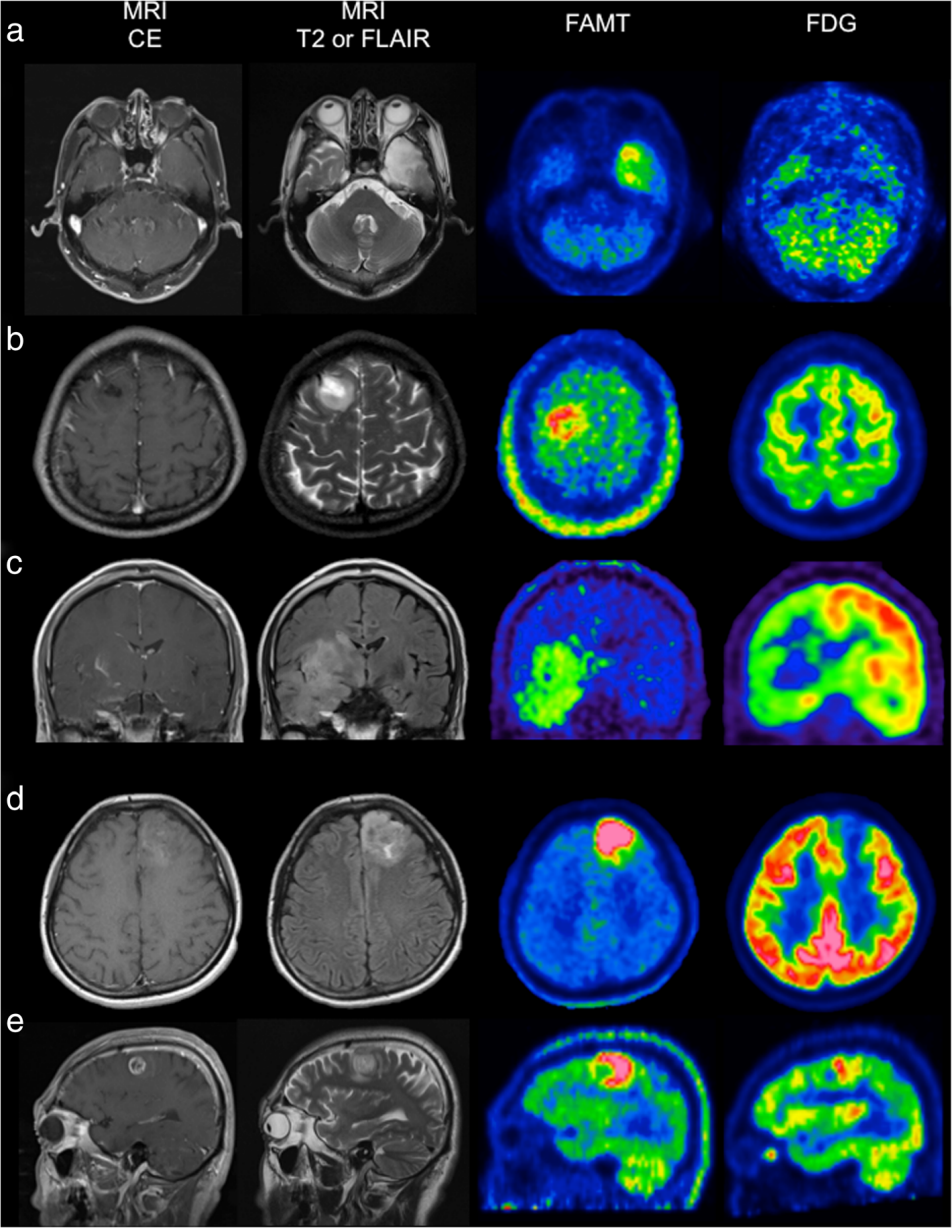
Representative cases. Contrast-enhanced T1-weighted magnetic resonance images (*MRI CE*), T2-weighted or fluid attenuated inversion recovery images (*MRI T2 or FLAIR*), ^18^F-FAMT PET images, and ^18^F-FDG PET images, *from left to right*. a A 71-year-old man with diffuse astrocytoma. ^18^F-FAMT T/N ratio was 2.05. ^18^F-FDG T/N ratio was 0.90. b A 32-year-old man with oligoastrocytoma. ^18^F-FAMT T/N ratio was 2.80. ^18^F-FDG T/N ratio was 0.86. c A 50-year-old man with anaplastic astrocytoma. ^18^F-FAMT T/N ratio was 5.33. ^18^F-FDG T/N ratio was 1.22. d A 39-year-old woman with anaplastic oligoastrocytoma. ^18^F-FAMT T/N ratio was 5.48. ^18^F-FDG T/N ratio was 0.58. e A 56-year-old man with glioblastoma. ^18^F-FAMT T/N ratio was 4.73. ^18^F-FDG T/N ratio was 1.05
